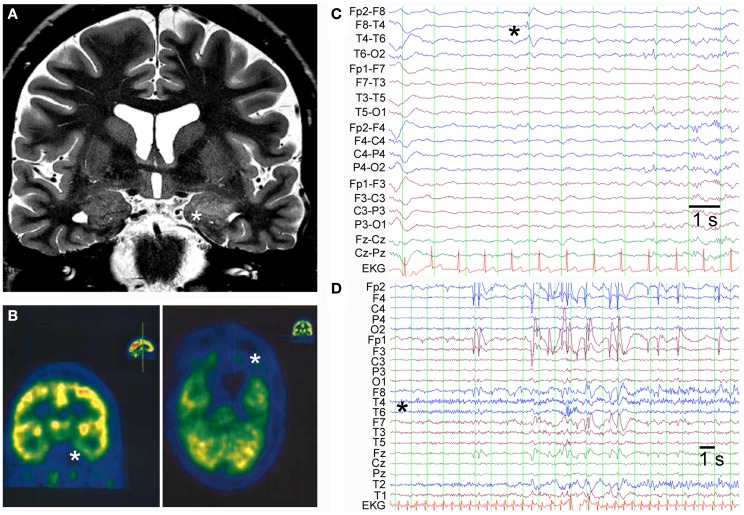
(A) Coronal T2-weighted cerebral MRI shows increased volume and signal in the left amygdala and anterior part of the hippocampus (indicated by asterisk). (B) FDG-PET displays hypometabolism in both temporo-mesial regions with a predominant deficit in the left hemisphere (indicated by asterisks: left panel coronal section and right panel axial section). (C) Interictal scalp EEG in bipolar montage shows sharp-slow-wave activity in the right fronto-temporal region (indicated by asterisk). (D) Ictal scalp EEG of a typical temporal lobe seizure demonstrates seizure-onset over the right temporal region with a regional maximum at the electrodes T6, T4, and T2 (unipolar montage against common average).
